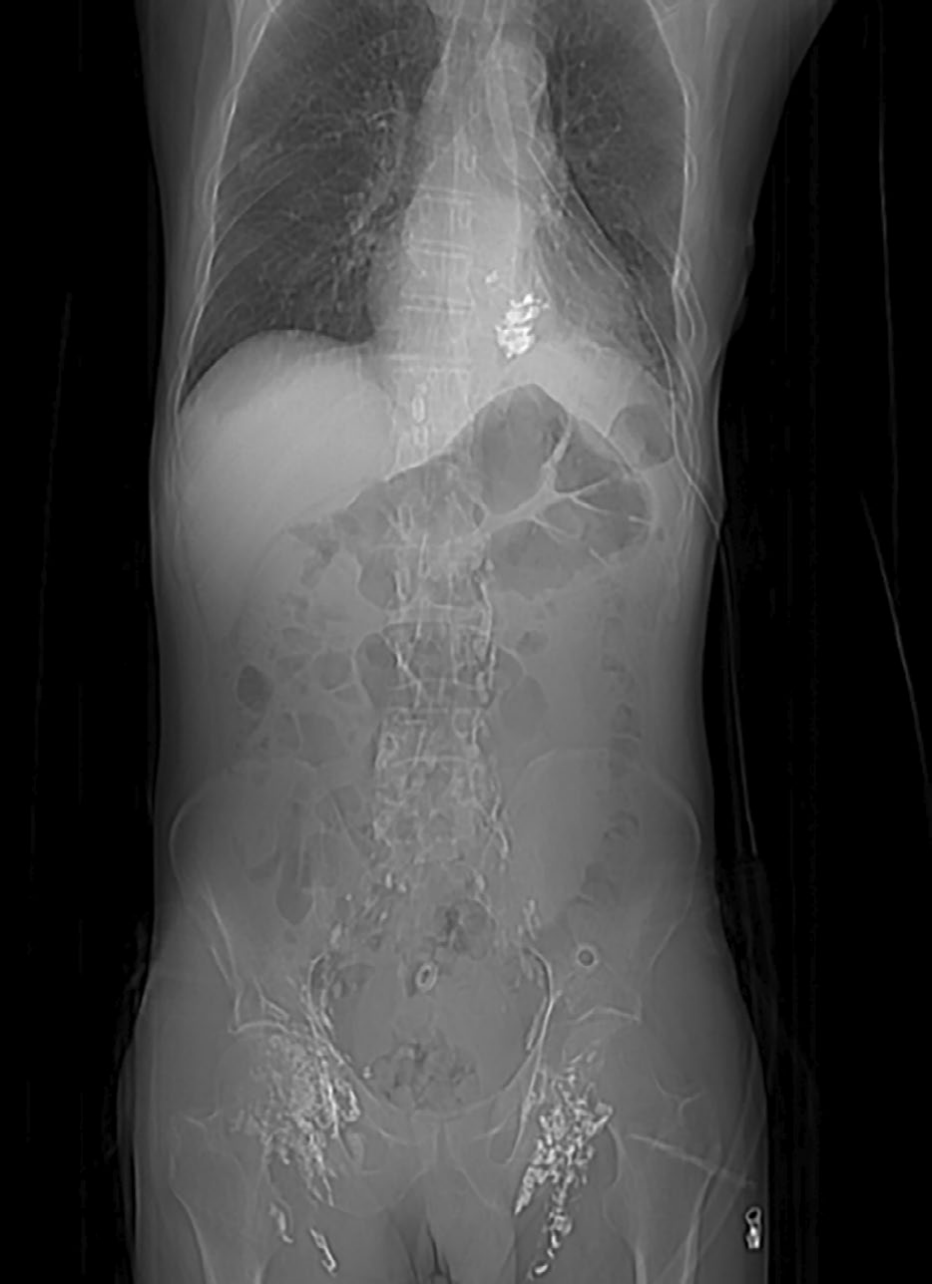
Evidence of contrast agent accumulation in the left pleural cavity and along the lymphatic chain from the inguinal lymph nodes, as detected through a chest CT scan.
